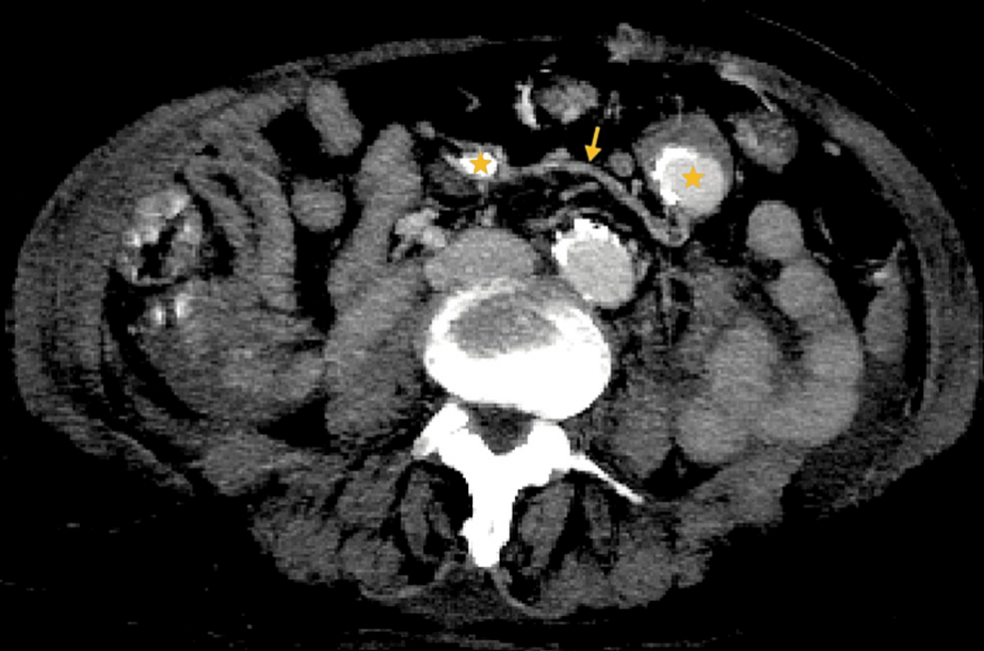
Abdominal CECT axial plane, in portal-venous phase and with positive-oral contrast administration. Yellow stars point to two saccular images with peripheral calcifications, close to the greater curvature of the stomach and in vascular trajectory (yellow arrow). CECT: Contrast-Enhanced Computed Tomography
